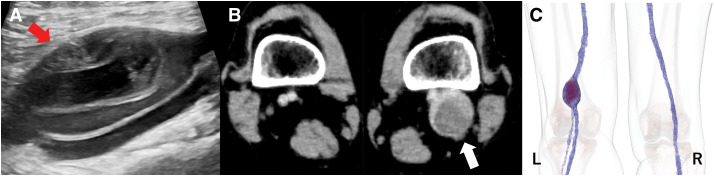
Fig. 1 (A) Echocardiography of the veins in the lower extremities revealed a venous aneurysm in the left popliteal fossa (red arrow). (B) Contrast-enhanced CT scan showed that the venous aneurysm was 60 mm in diameter and filled with thrombus (white arrow). (C) Three-dimensional CT image showed a fusiform-type popliteal venous aneurysm. The image is a dorsal view of the PVA, with the thrombus in the PVA shown in dark purple.
